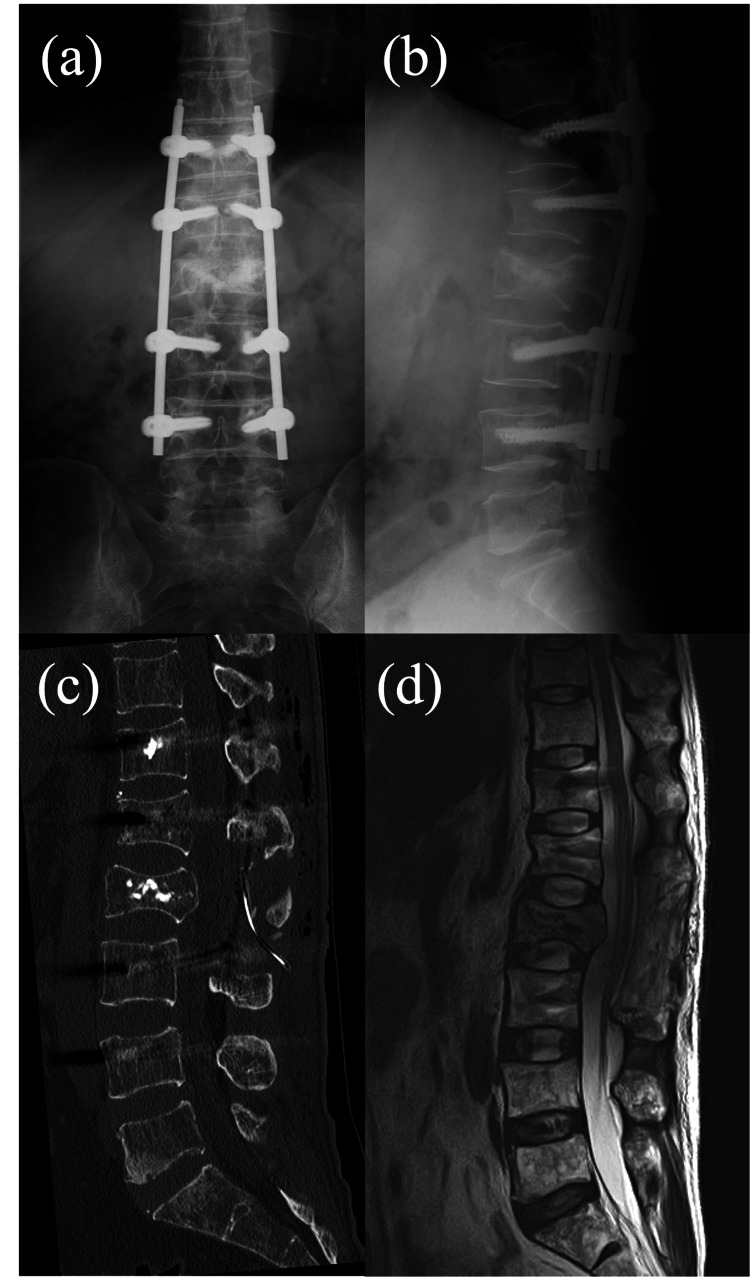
Postoperative images. (a, b) Postoperative X-rays of the thoracolumbar spine. (c, d) Sagittal CT and MRI T2-weighted imaging.
